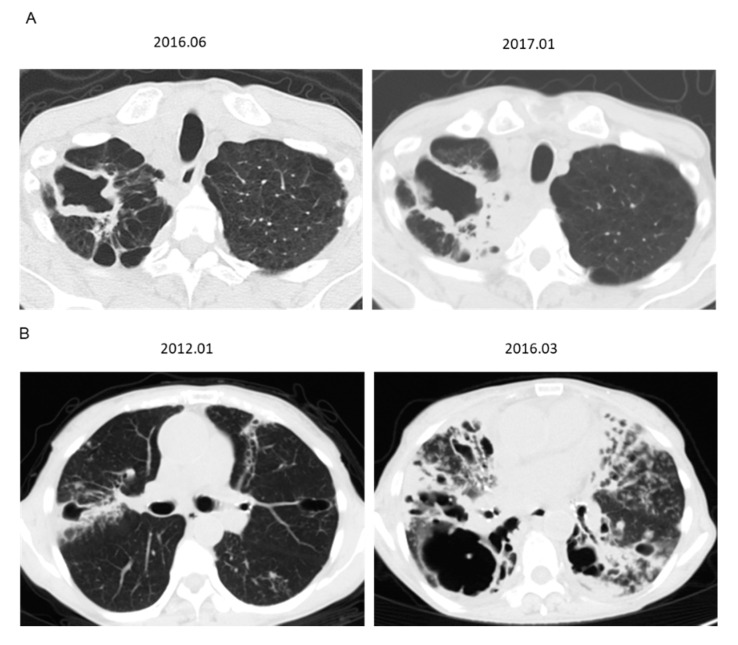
Serial computed tomography scans of patients with cavitary *Mycobacterium avium* complex pulmonary disease (MAC-PD) before and after diagnosis of chronic pulmonary aspergillosis (CPA). (A) Left: at the time of MAC-PD diagnosis (fibrocavitary form). *Aspergillus* galactomannan antigen 0.3, β-D glucan 8.8. Right: at the time of CPA diagnosis. *Aspergillus* galactomannan antigen 0.4, *Aspergillus* precipitating antibody-positive, β-D glucan 18.8 pg/mL, and isolation of *A. fumigatus* from sputum culture. (B) Left: at the time of MAC-PD diagnosis (cavitary nodular bronchiectasis). *Aspergillus* galactomannan antigen 0.2, β-D glucan 7.5. Right: at the time of CPA diagnosis. *Aspergillus* galactomannan antigen 5.0, *Aspergillus* precipitating antibody-positive, β-D glucan 300.0 pg/mL, and isolation of *A. fumigatus* from sputum culture.
